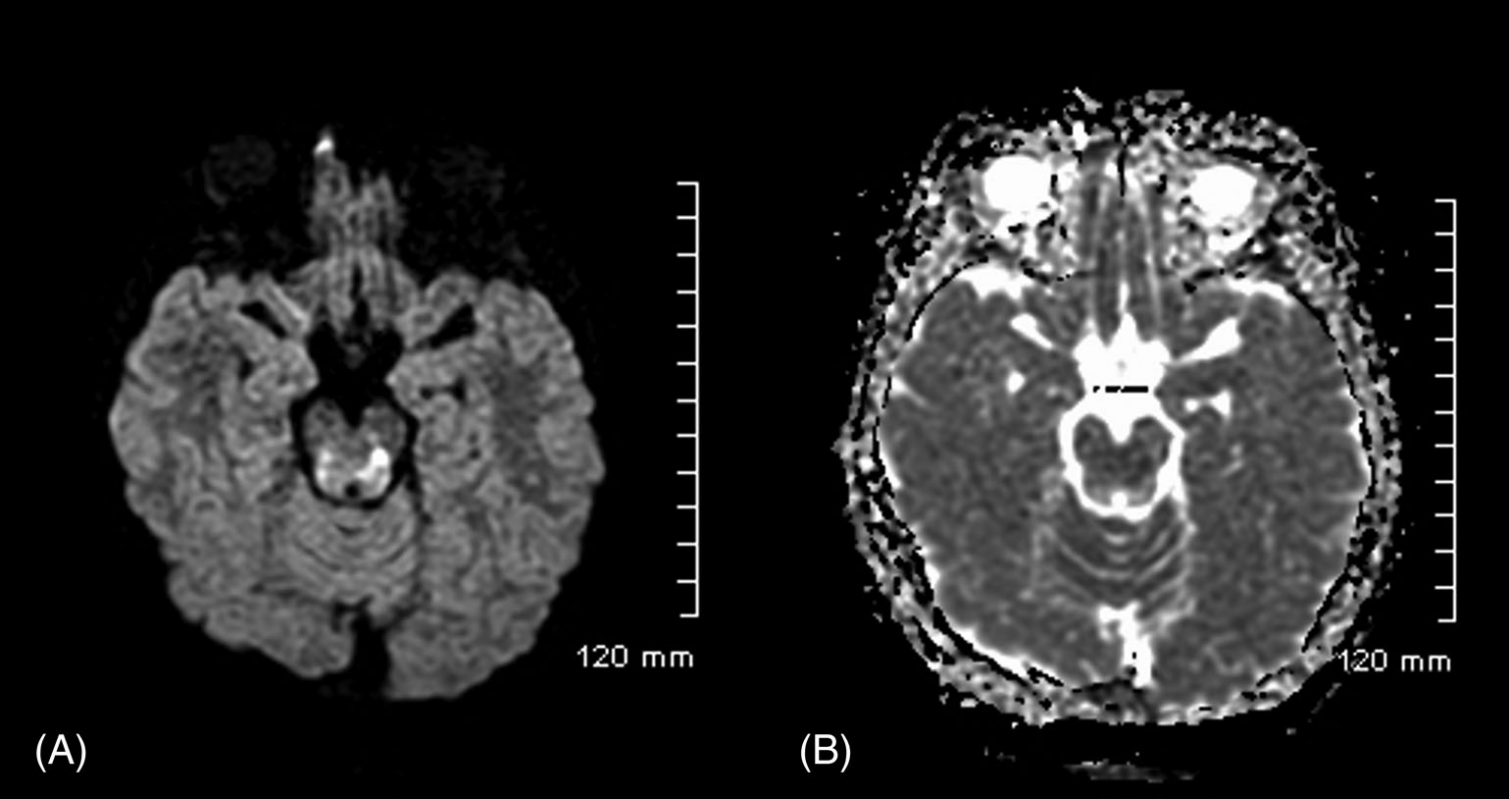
Magnetic resonance imaging of the brain on day 2 of admission showing diffusion‐weighted imaging (DWI) and apparent diffusion coefficient (ADC) mapping. Imaging obtained at 9 years and 7 months of age. Patchy reduced diffusivity seen within the midbrain on (A) axial DWI and (B) axial ADC map.
